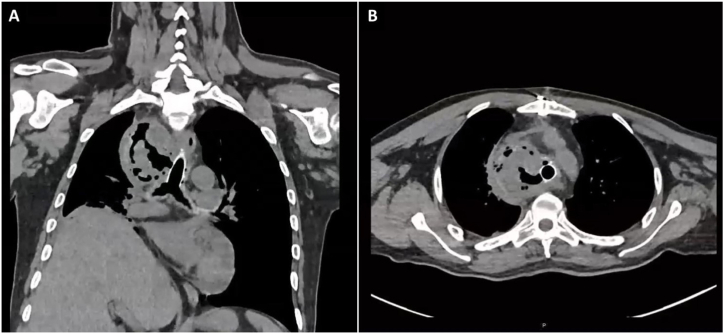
Frontal section computed tomography (A) and axial section (B): partially thrombosed brachiocephalic trunk aneurysm with hydroaeric level and trachea fistulization contained by silicone stent wall was observed.
